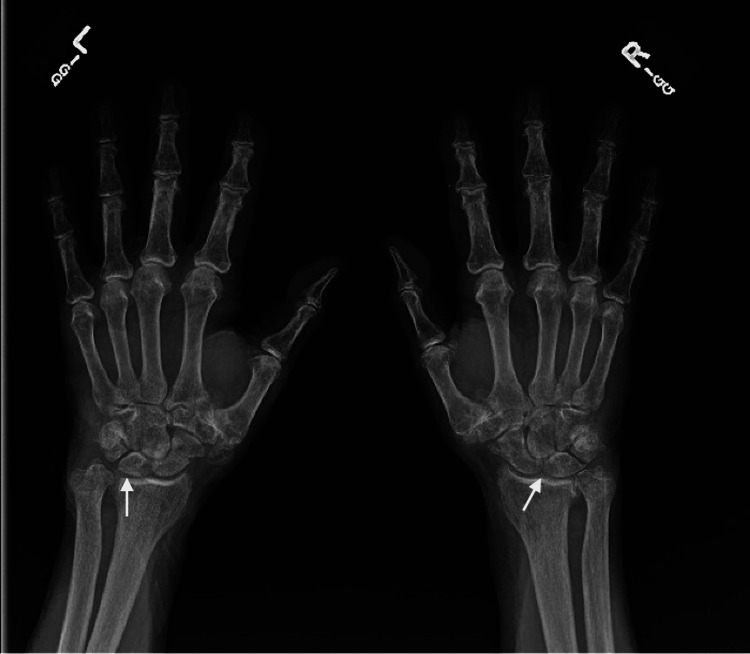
Plain radiograph of the hands Plain radiograph of the hands shows diffuse osteoarthritis and radiocarpal chondrocalcinosis (noted by arrows)
